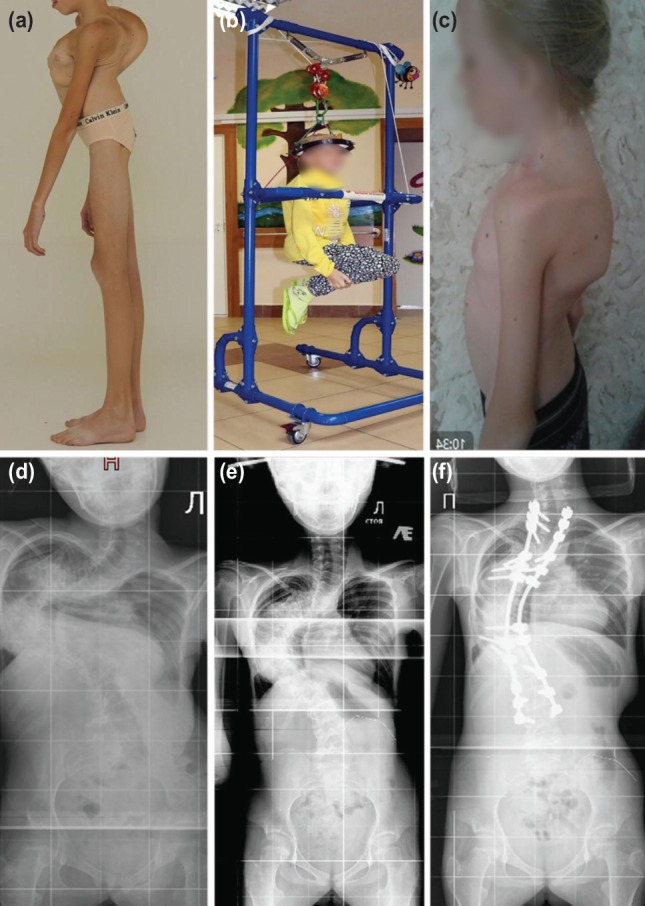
13-year old with Ehlers-Danlos syndrome (Case 2). Images (a) pre-operative, (b) on traction and (c) post-operative. Radiographs (d) pre-operative, (e) on traction and (f) post-operative.
